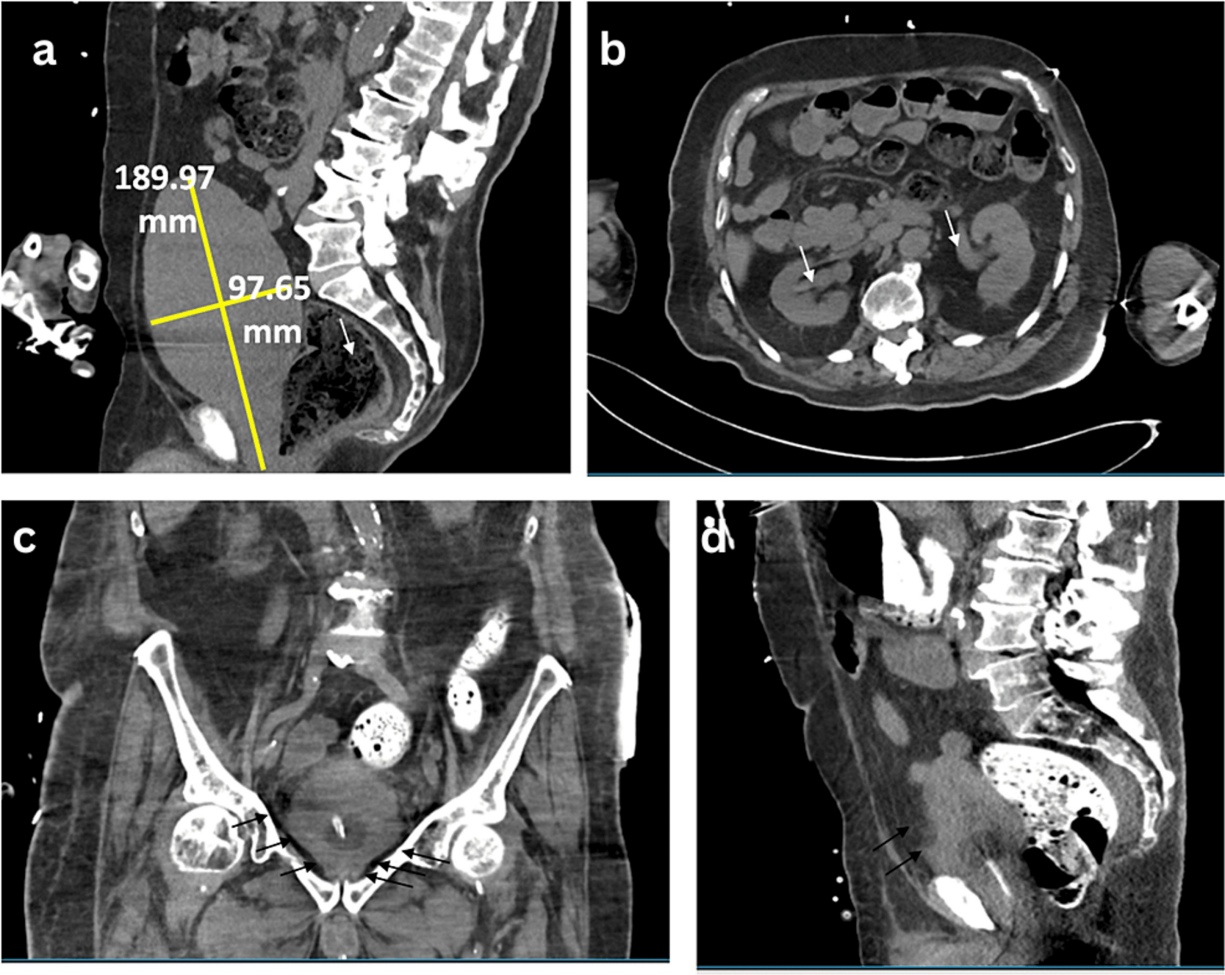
CT pelvic scan showing distended and conical configuration bladder with emphysema cystitis and stool impaction. a: The bladder appears distended with a conical configuration. There is funneling of the bladder neck with mild bilateral hydronephrosis. b: Bilateral hydronephrosis (white arrow). It suggests a neurogenic bladder and bladder outlet obstruction with obstructive nephropathy. The lower panels of the CT pelvic scan (c and d) show emphysema cystitis with markedly abnormal bladder. The thickening bladder wall contains gas (black arrows), suggesting emphysematous cystitis (bottom left, coronal view; bottom right, sagittal view).
